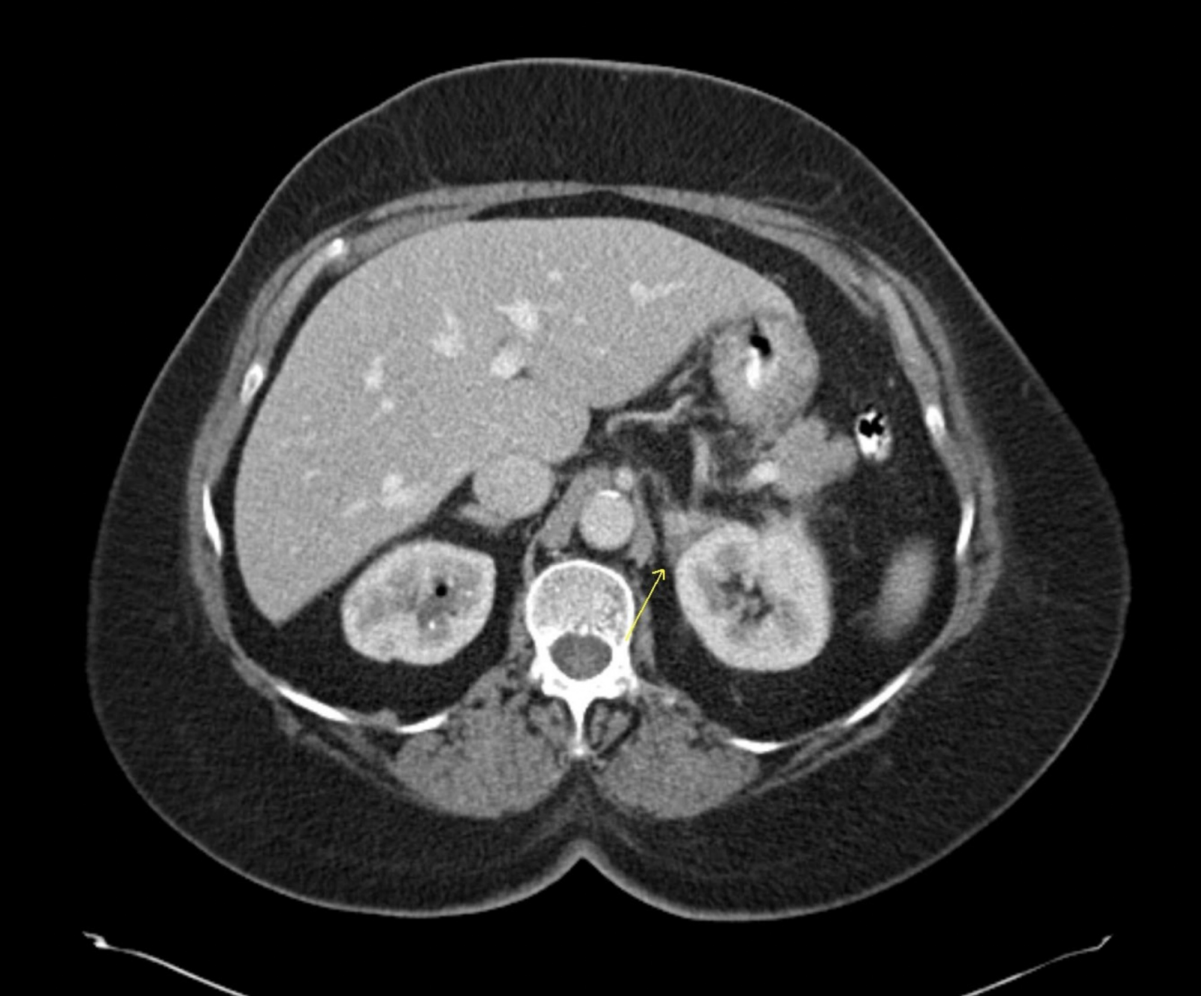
CT of the abdomen W/contrast showing decrease in soft tissue thickening of the suprarenal mass (arrow).
